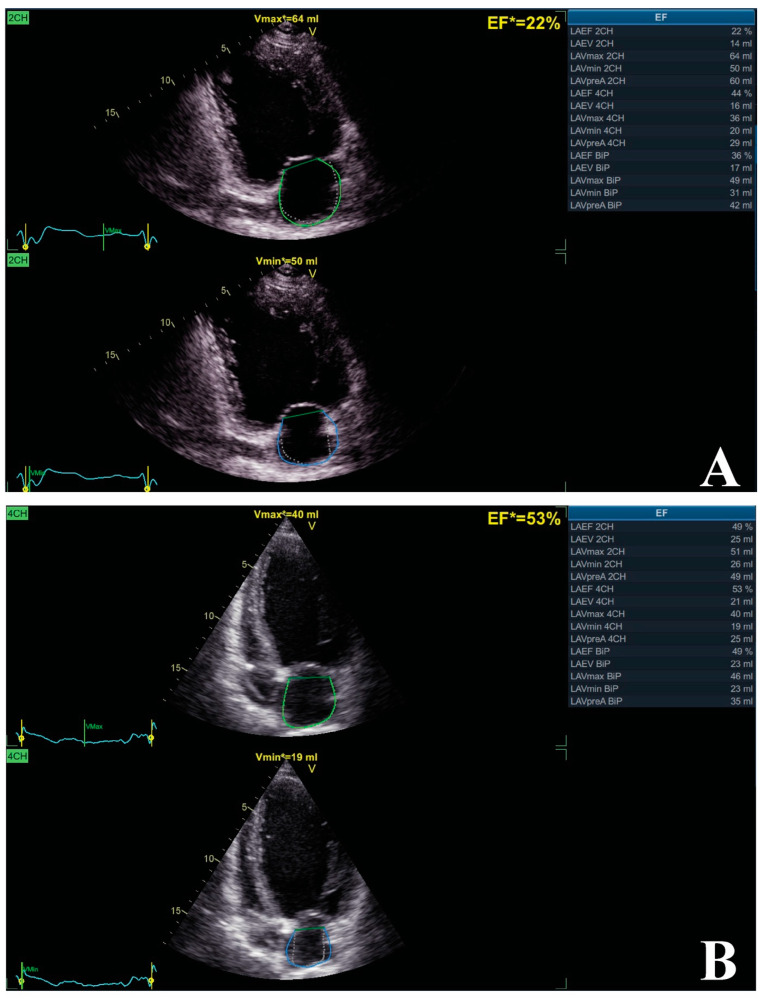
The impact of CRT on LA volumes. Patient with dilated cardiomyopathy, LVEF of 33%, and QRS morphology of LBBB with duration of 153 msec. Echocardiographic evaluation using a General Electric VIVID E95 vendor and EchoPac software package to determine LA biplane volumes (Images from the personal collection of the authors). Initial evaluation showed increased LA volumes (A) and, after a 6-month follow-up, significant LA remodeling with reduction in LA volumes can be seen (B). Abbreviations: LA, left atrium; LBBB, left bundle branch block; LVEF, left ventricle ejection fraction.
